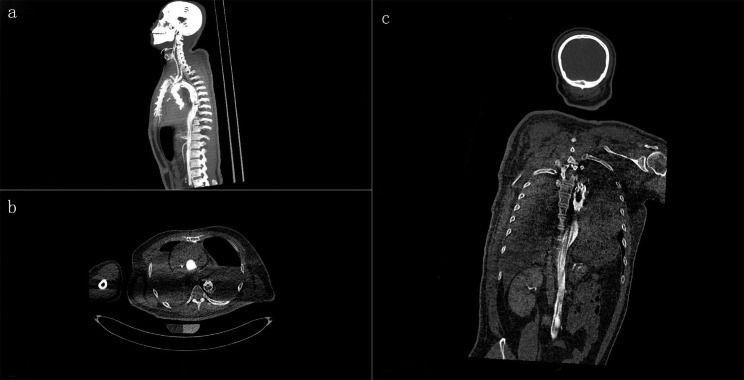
Radiological findings. The postmortem computed tomography angiography (PMCTA) results show no flow of the contrast agent from the blood vessel in the (a) sagittal, (b) axial, or (c) coronary aspect
